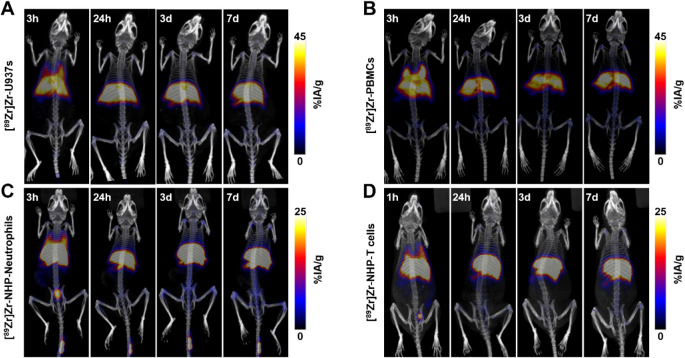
Representative maximum intensity projections (MIPs) of PET/CT images
